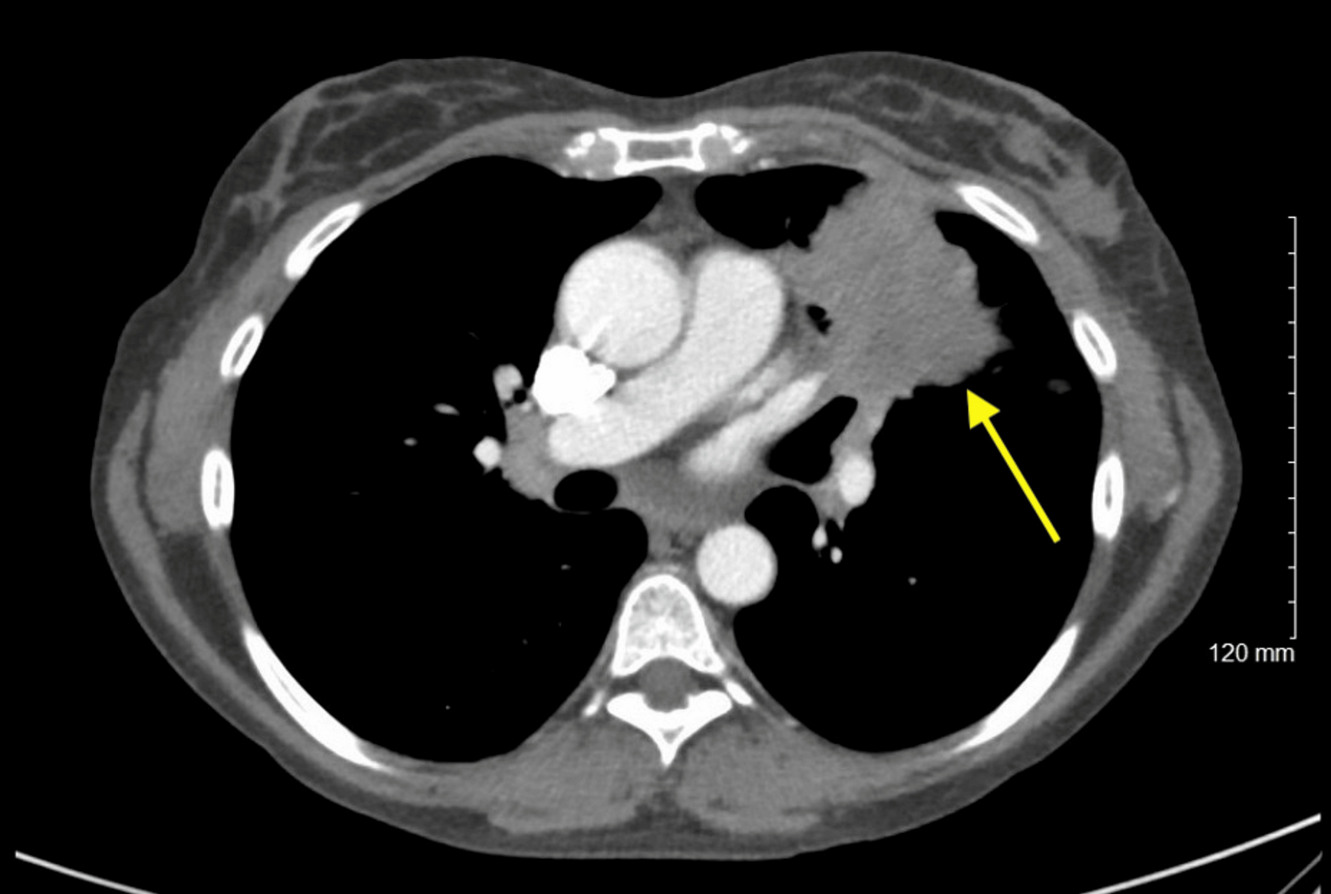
Axial CT of the chest with contrast showing the left upper lobe lung mass with mediastinal invasion
